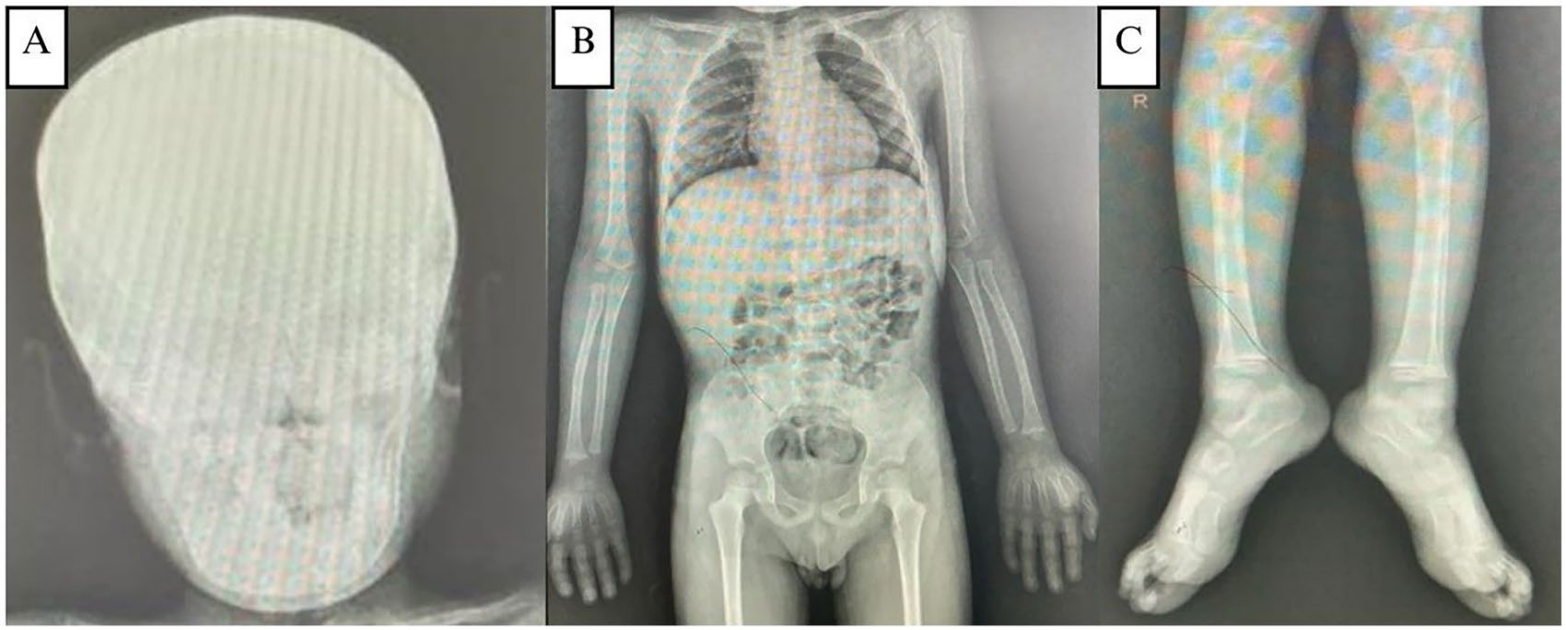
(A, B, and C) X-ray of the skull reveals calcification along with good bone trabeculation, inhomogeneous opacity in the mandibular area, an amorphous form, and distinct borders with regular edges. Both the pedicles and the corpus are intact. There are no apparent osteolytic or osteosclerotic lesions. An X-ray of the lower, upper, and pelvic extremities shows that there is strong trabeculation and bone structure. There is no apparent osteolysis or osteosclerosis.
